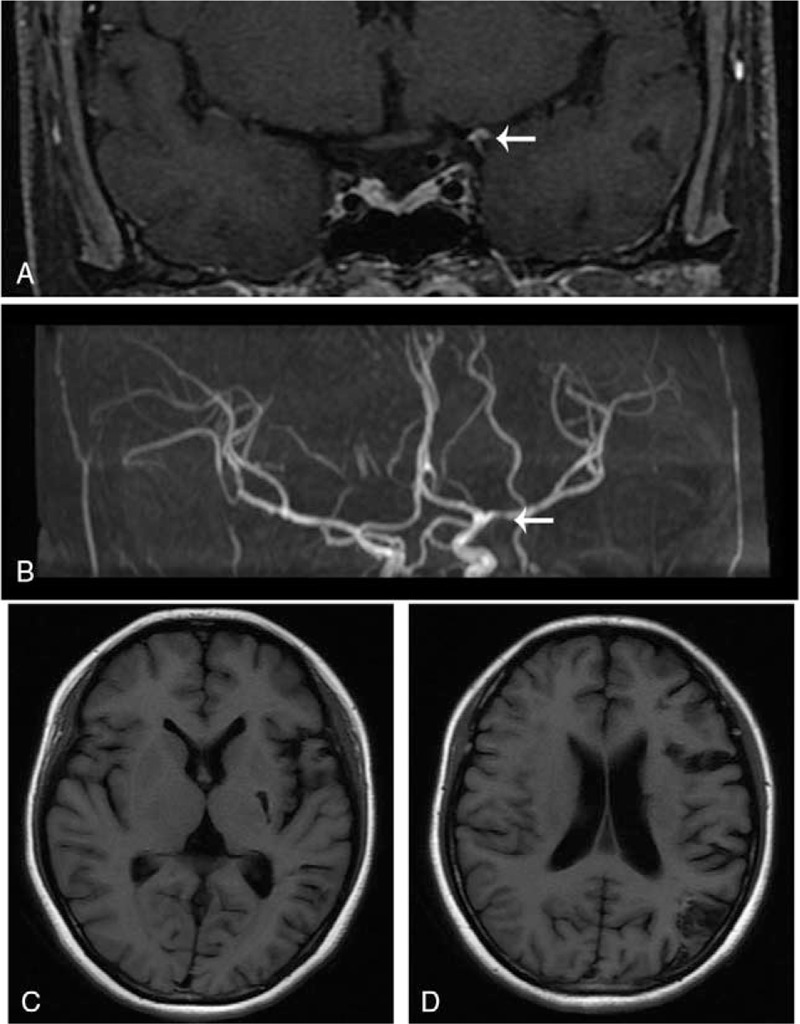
The MR image after MT. A, On the thin slice contrast-enhanced MR an obvious enhancement of vessel wall at left M1 segment was detected. B, MRA revealed stenosis at the same site. C and D, her T1 weighted MR showed multiple encephalomalacia foci 4 months later.
